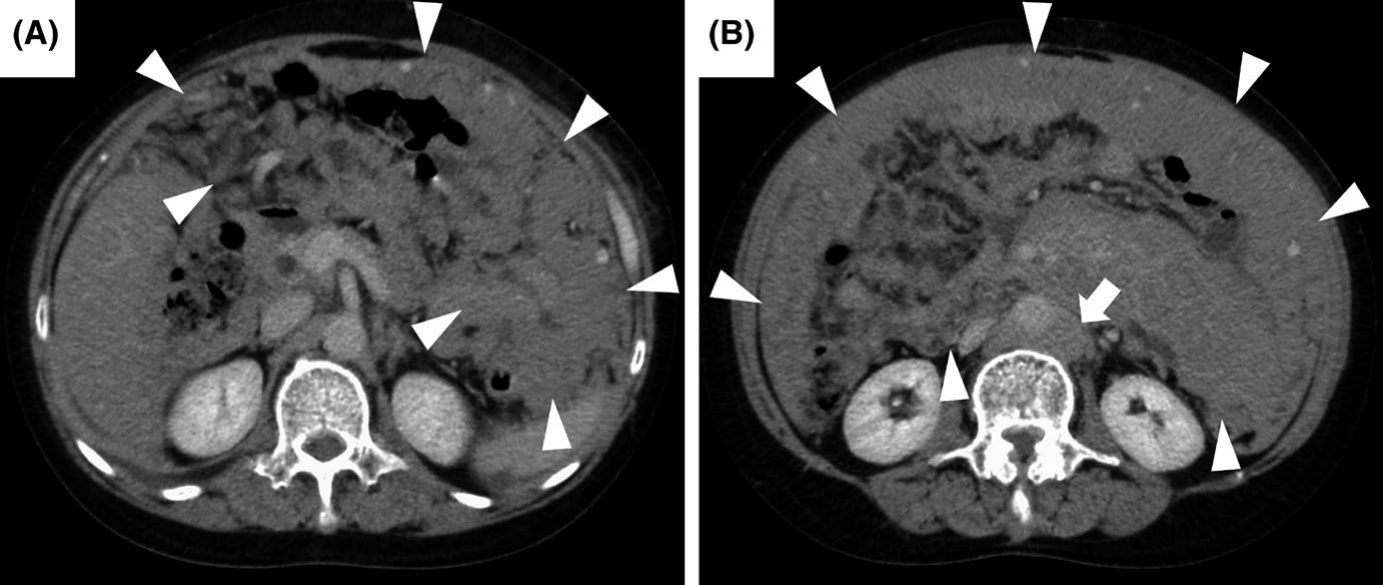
Chest and abdominal computed tomography with contrast enhancement. Chest and abdominal computed tomography with contrast enhancement revealed multiple fused and homogeneously contrasting masses filling the abdominal cavity (A, B: arrowheads) and para‐aortic lymph nodes (B: arrow)
